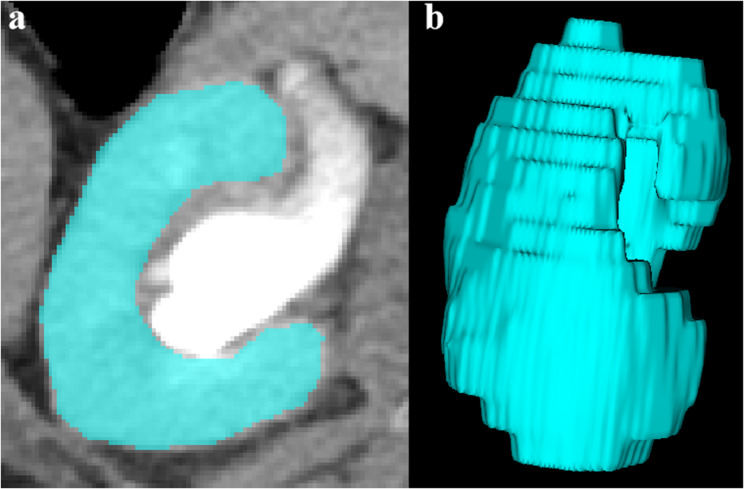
Renal volume of interest (VOI) on excretory phase CT. (a) Axial image at the renal hilum; (b) 3D representation of the segmented VOI
